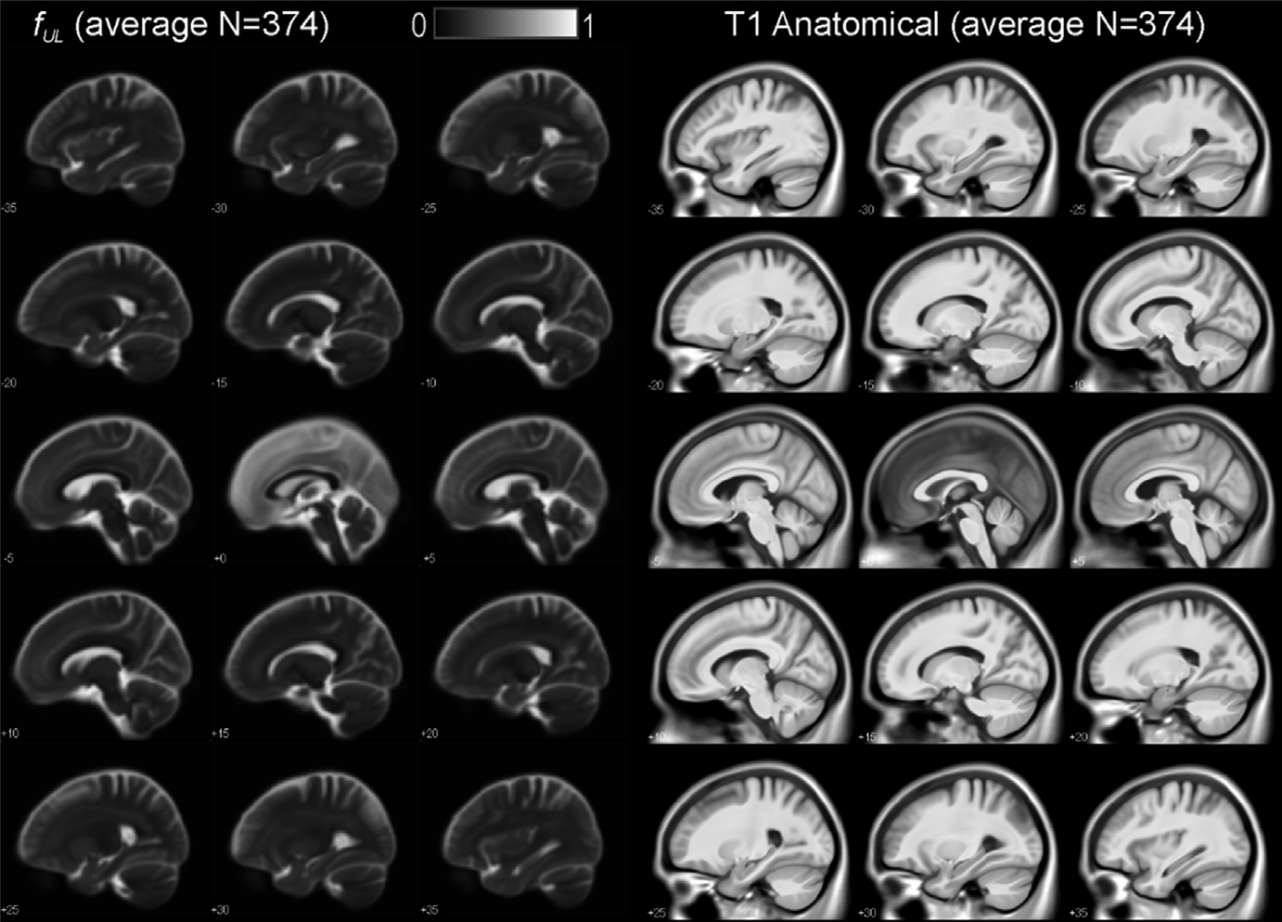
Average *f*_*UL*_ free water fraction upper limit and corresponding anatomical T1-weighted scans for 375 IXI subjects. Locations of sagittal slices are in x-values in MNI space.
